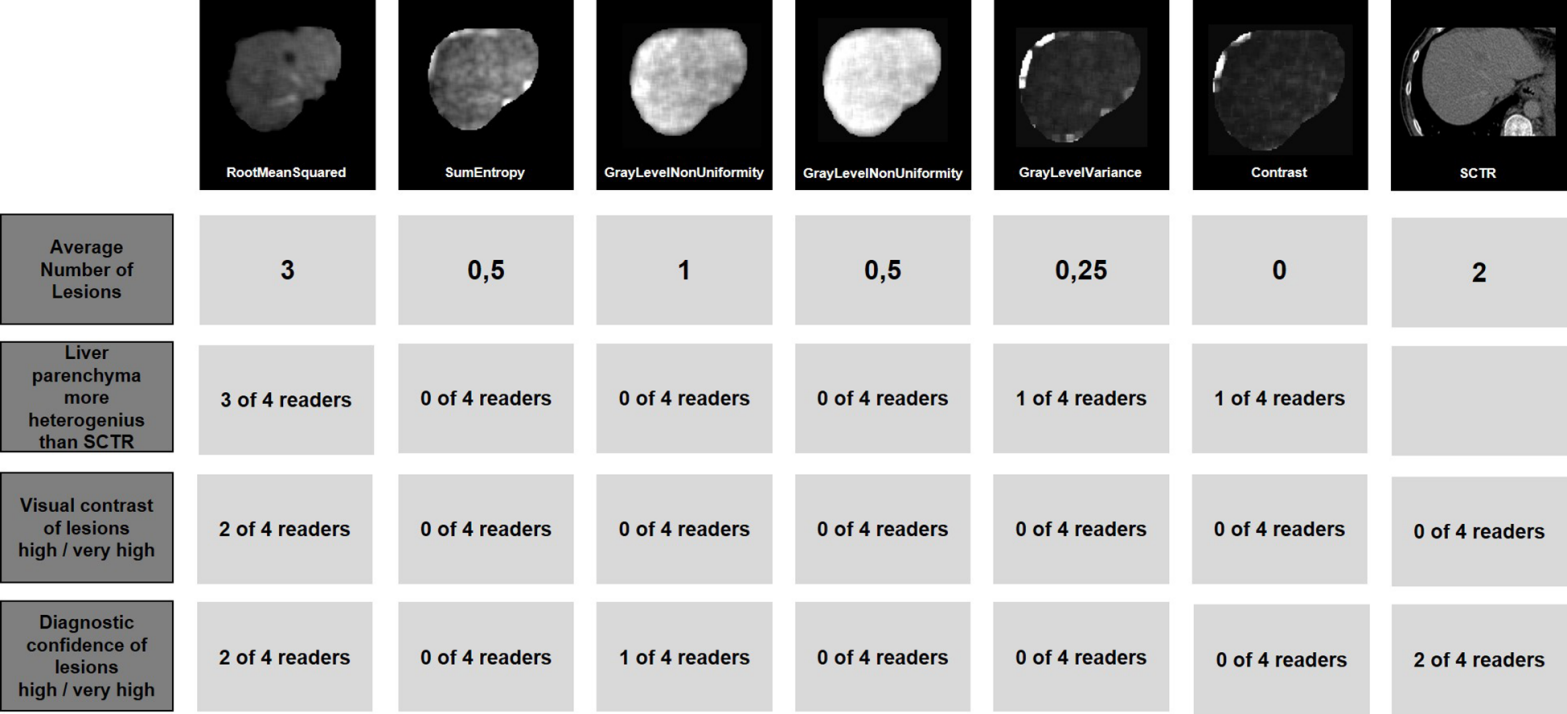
Example reading of one patient. The image section of an exemplary lesion is shown in the standard CT reconstruction as well as in all evaluated radiomics maps. In addition, the Average number of lesions is shown as well as the number of readers who rated the liver parenchyma as more heterogeneous than in the SCTR, the visual contrast as high or very high, and the diagnostic confidence as high or very high. Original firstorder RootMeanSquared shows a superior contrast for two liver lesions.
